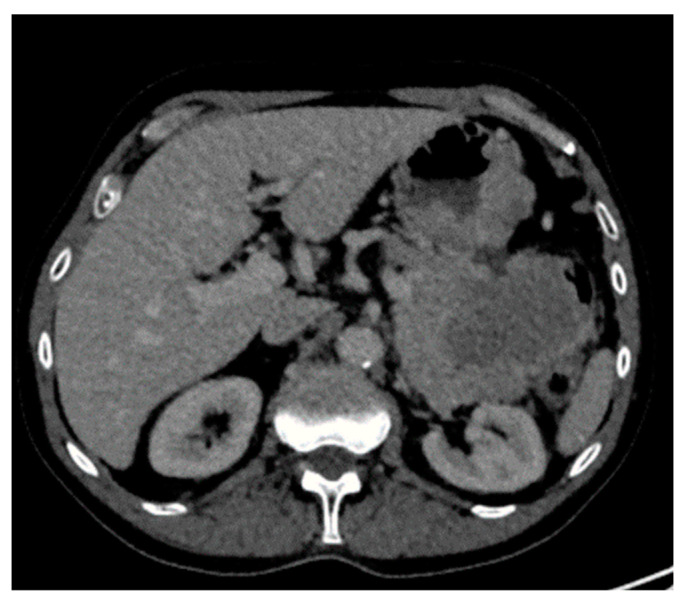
Biopsy-proven Gastric EP, case from our institution (Axial contrast-enhanced CT image shows a heterogeneous enhancing gastric mass with exophytic and intraluminal components.
